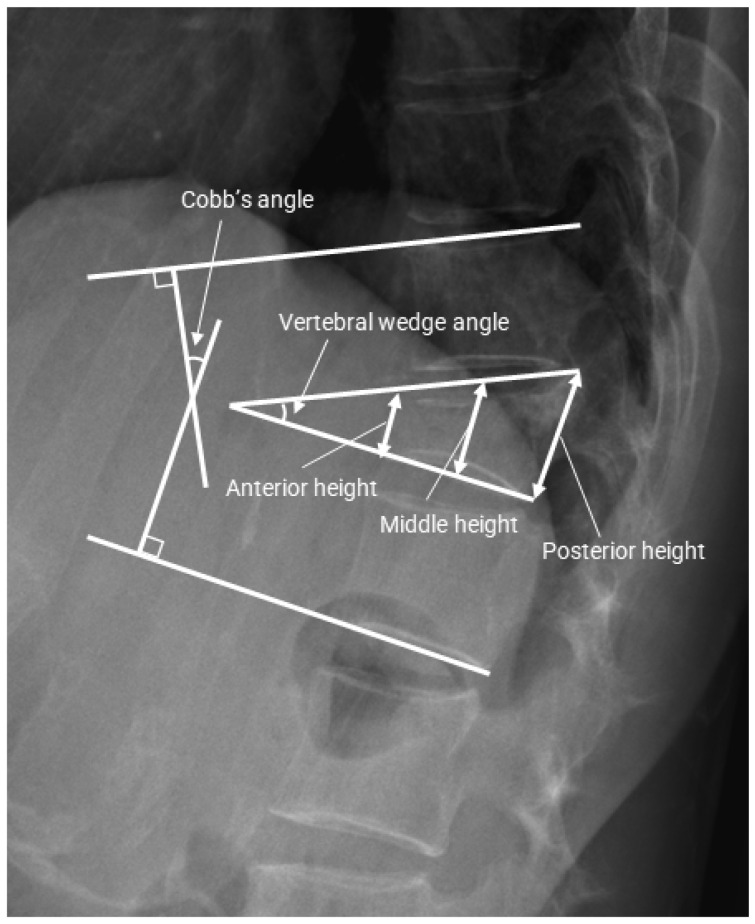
Radiographic parameters used for assessment. Cobb angle (CA), vertebral wedge angle (VWA), anterior vertebral height (AVH), middle vertebral height (MVH), and posterior vertebral height (PVH).
